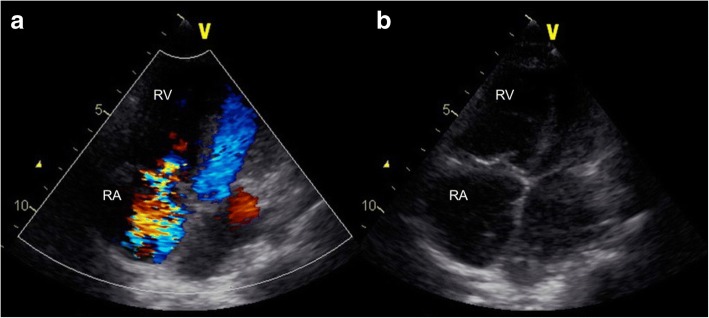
Echocardiogram in the apical view showing severe tricuspid regurgitation a: B-mode image, b: Color Doppler image. RV, right ventricle; RA, right atrium
